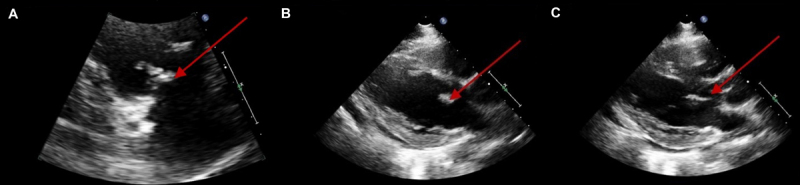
Transthoracic echocardiography of trivalvular vegetation. Vegetations observed on transesophageal echocardiography were as follows: (A) tricuspid valve (16 × 8.1 mm), (B) mitral valve (13.6 × 8.0 mm), and (C) aortic valve (18.3 × 4.7 mm).
